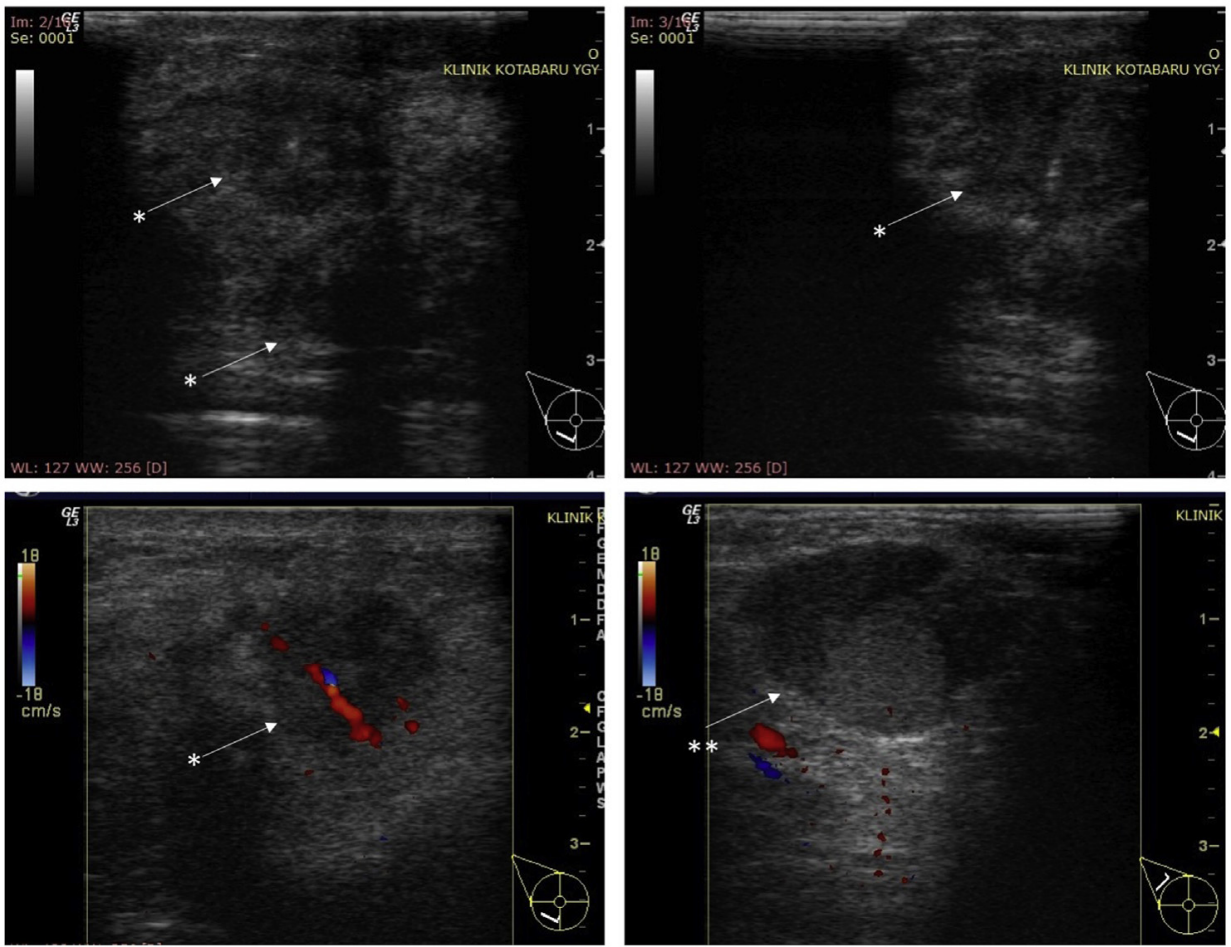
Ultrasonography of the right breast revealed diffuse hypo-echoid masses (*) with irregular border as well as enlargement of multiple lymph nodes in the right axillae ().
